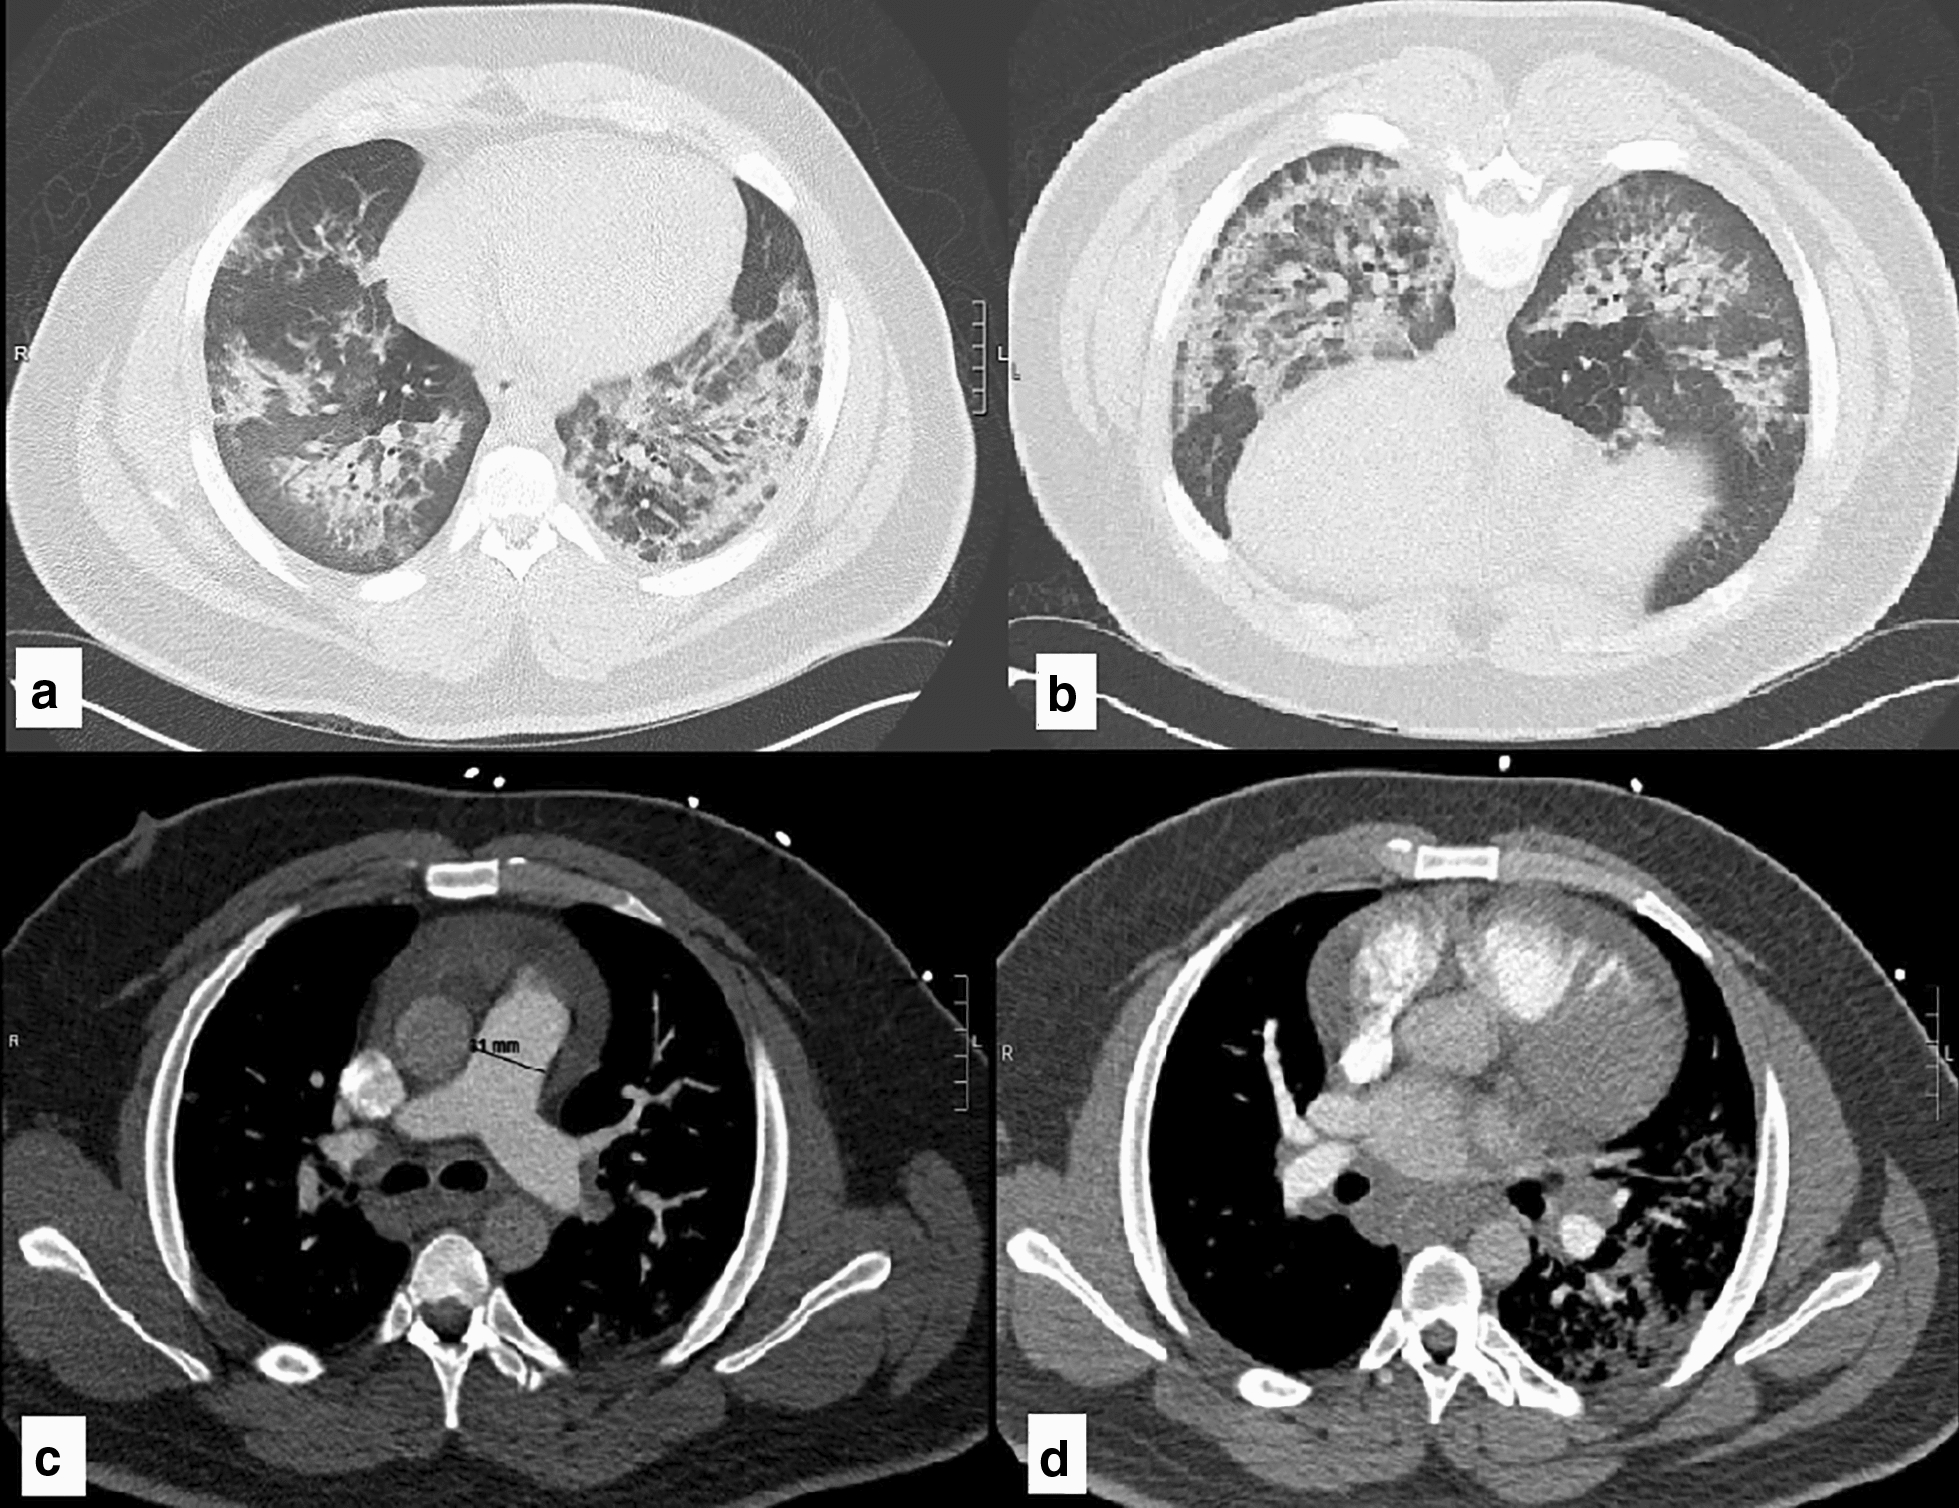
CT scans of the chest. a CT Scan of the chest on first day of presentation demonstrating bilateral centrilobular opacities, hilar/mediastinal lymphadenopathy, moderate cardiomegaly, a trivial pericardial effusion and enlargement of the main pulmonary artery. b Prone CT chest on day 7 of admission, revealing persistent intra-alveolar opacities with associated mediastinal lymphadenopathy
